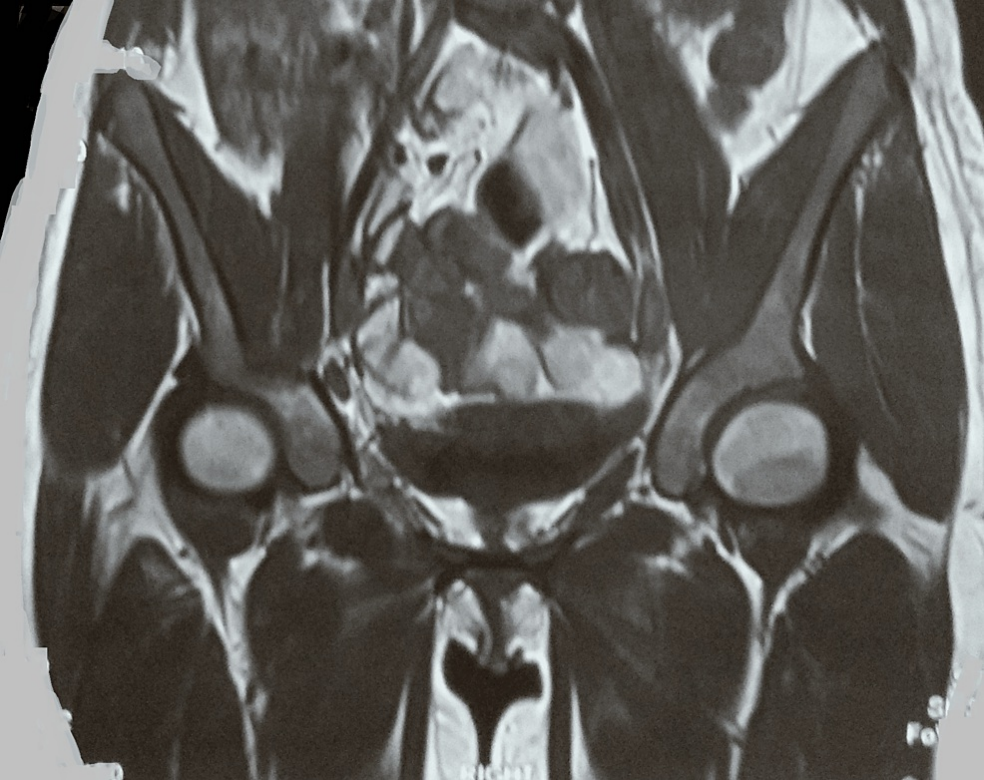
MRI of the right hip showing complete resolution of the disease MRI: magnetic resonance imaging
